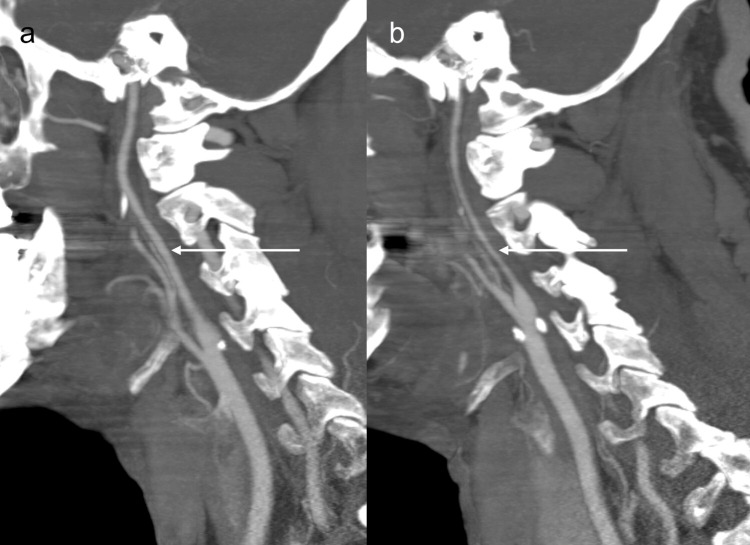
CTA sagittal maximum intensity projection (MIP) shows cervical internal carotid arteries (ICAs). (a) CTA sagittal MIP shows right cervical ICA without stenosis (arrow). (b) CTA sagittal MIP shows diffuse narrowing of left cervical ICA (arrow).
